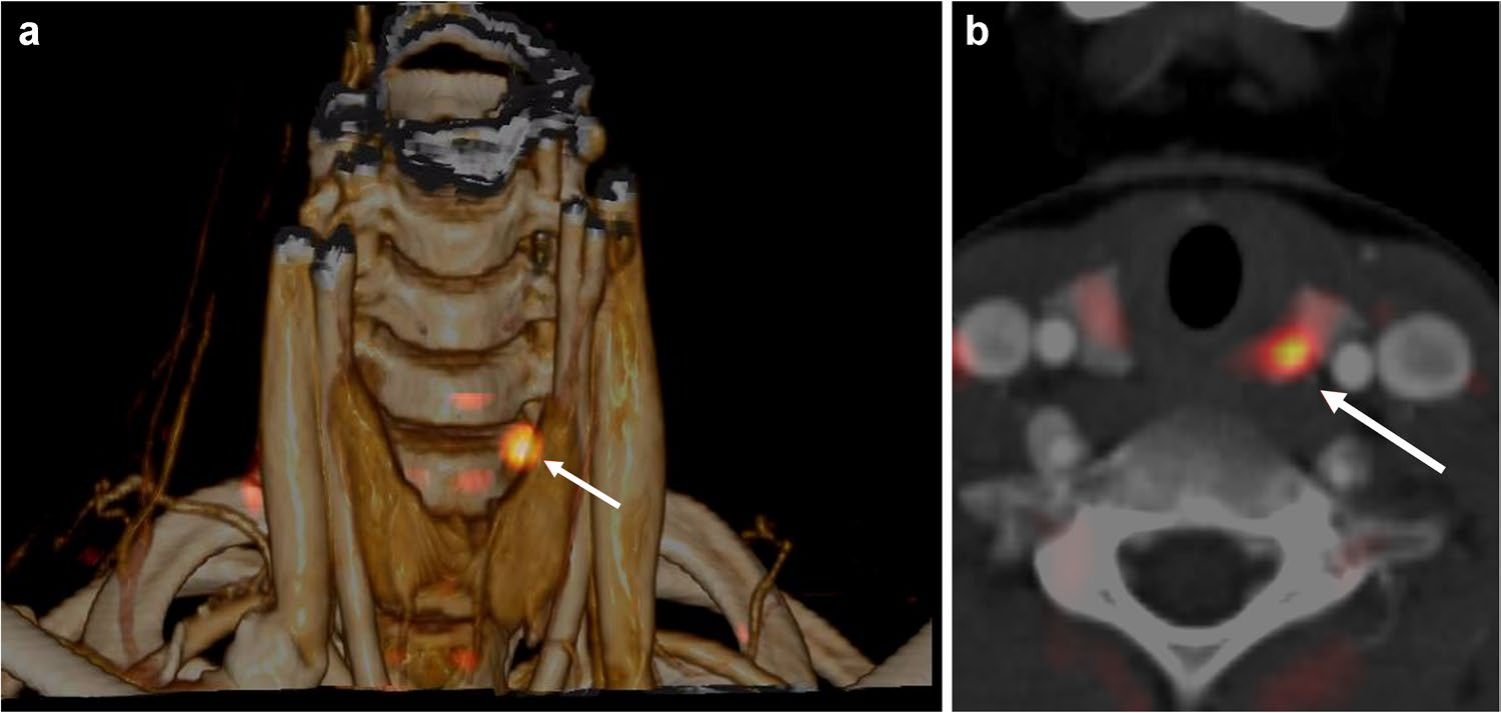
An 11-year-old male patient with diagnosis of primary hyperparathyroidism and history of kidney stones underwent [^18^F]fluorocholine positron emission tomography/computed tomography (PET/CT) to localize hyperfunctioning parathyroid gland(s) after negative or inconclusive first-line imaging methods (ultrasound and parathyroid scintigraphy using SPECT/CT acquisition). PET/CT was performed 45 min after the injection of 74 MBq of [^18^F]fluorocholine (field-of-view, 50 cm). Volume-rendered (a) and axial (b) [^18^F]fluorocholine PET/CT images detected an area of increased radiotracer uptake corresponding to a small nodule (maximum diameter, 8 mm) located behind the upper portion of the left thyroid lobe (*arrows*) highly suspicious for eutopic left superior parathyroid adenoma. Based on these PET/CT findings, the patient underwent left parathyroidectomy with excision of a pathology proven parathyroid adenoma. Primary hyperparathyroidism is a rare condition in children and treatment usually includes surgical removal of hyperfunctioning parathyroid gland(s). The figure demonstrates that [^18^F]fluorocholine PET/CT may be an effective imaging method to detect and localize hyperfunctioning parathyroid glands in pediatric patients with primary hyperparathyroidism.
